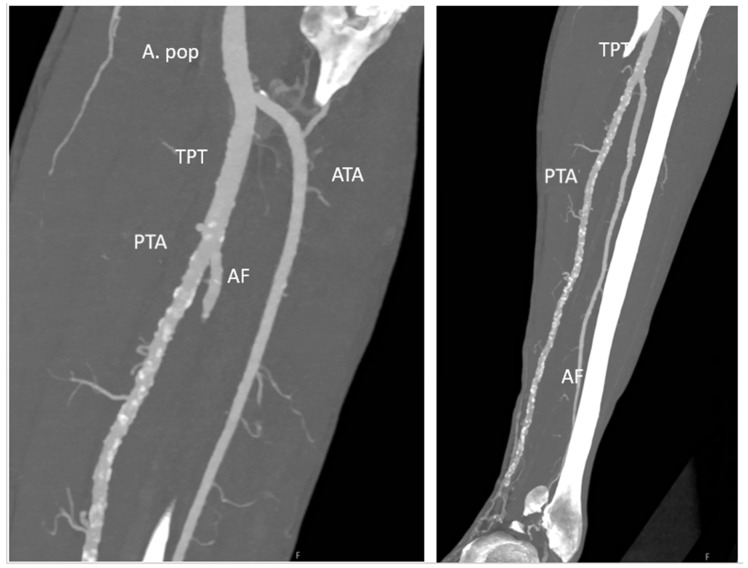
Lower limb vascular assessment using photon-counting CT (PCCT). Coronal maximum intensity projection (MIP, 10 mm) of the left lower extremity acquired with PCCT. The popliteal artery (A. poplitea), anterior tibial artery (ATA), posterior tibial artery (PTA), tibioperoneal trunk (TPT), and fibular artery (AF) are visualized, enabling preoperative donor-site vascular evaluation within a single imaging acquisition.
